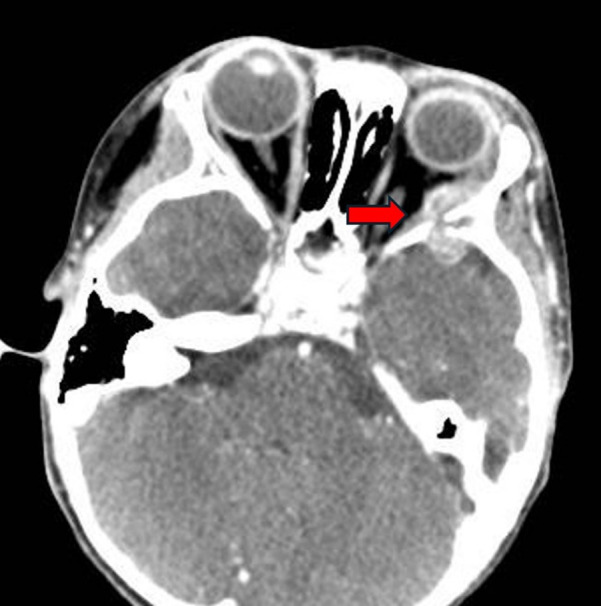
Radiographic findings of tumor progression. Contrast-enhanced CT performed 3 months after completion of adjuvant therapy showing progression of the lesion, with extension beyond the lateral wall into the left infratemporal fossa and the left temporal lobe (arrow)
